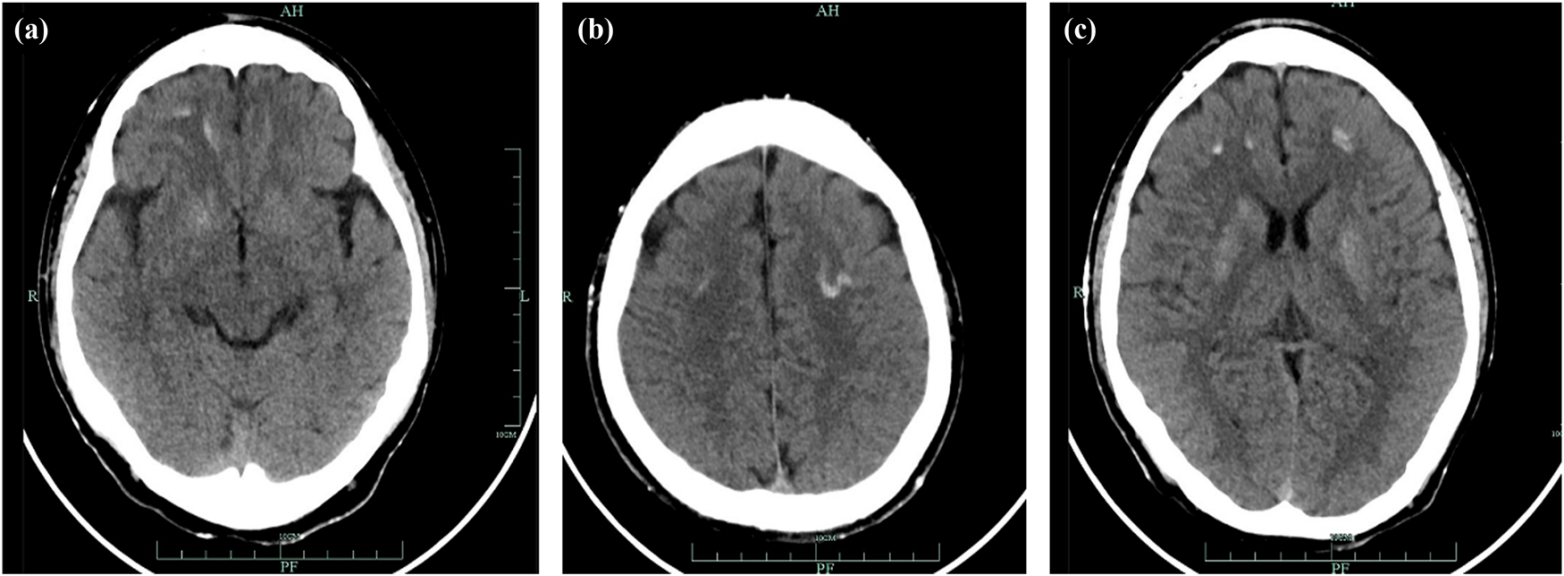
The head CT scan image (a–c) of the patient showed multiple calcifications.
